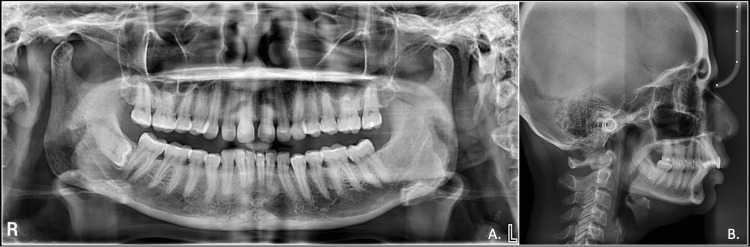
Pre-treatment radiographs A: OPG; B: lateral cephalogram OPG: orthopantomogram
